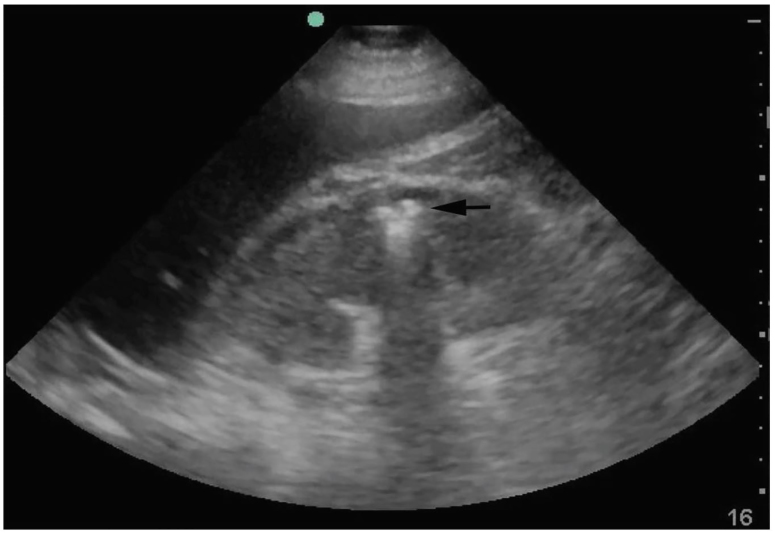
Point-of-care ultrasound demonstrating emphysematous pyelonephritis. In this still image, air can be seen in the renal cortex as a hyperechoic focus (black arrow) with posterior “dirty shadowing” as a result of reverberation artifact.
